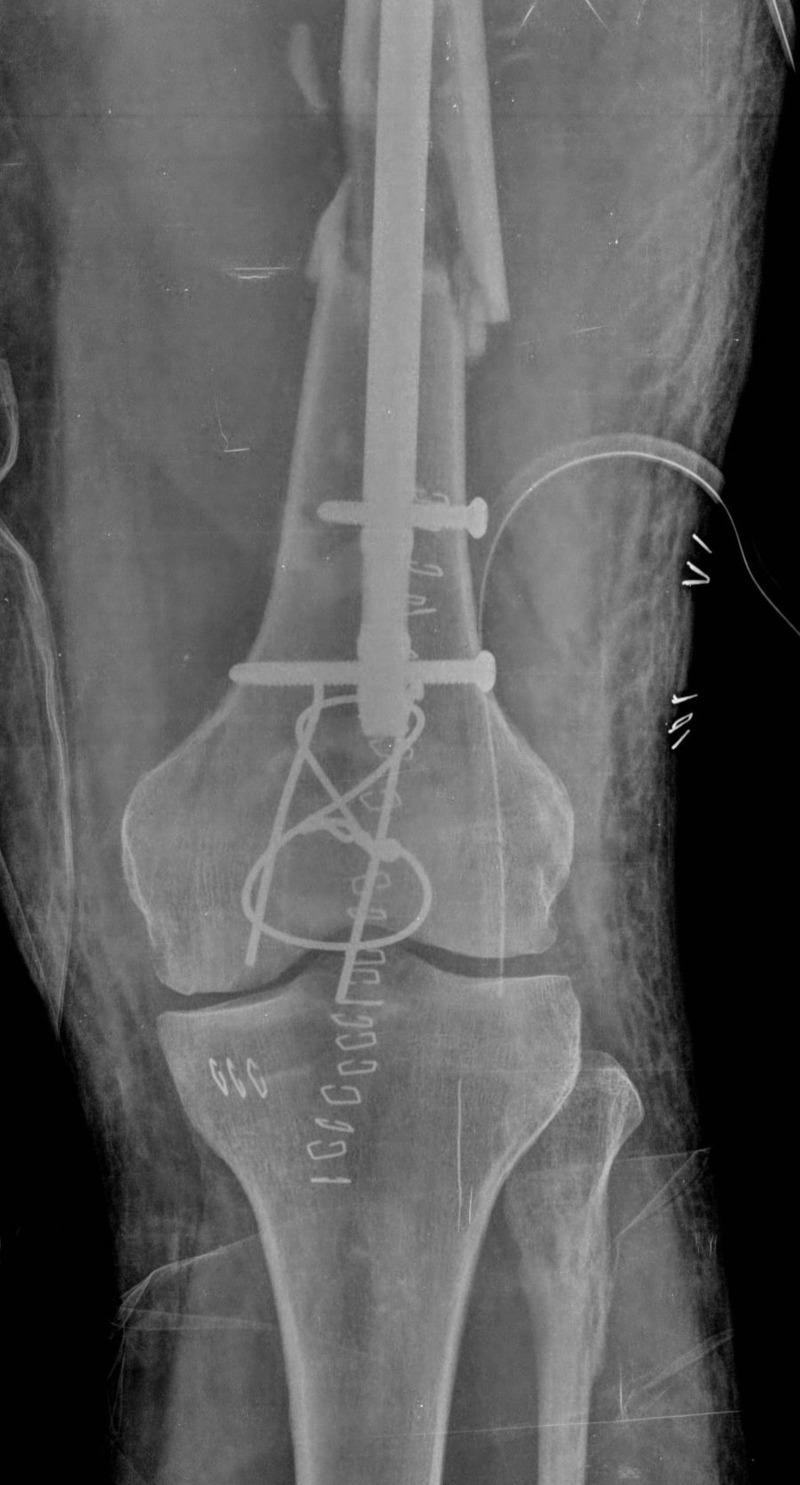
Immediate post-op antero-posterior x-ray of the patellar fracture with tension band wiring and femur interlocking
